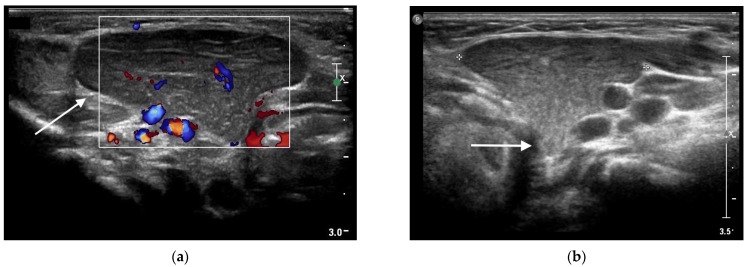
Ectopic cervical thymus in a 11-month-old toddler accidentally noticed on clinical examination for an acute upper respiratory infection. (a) Longitudinal sonogram showing a 3.5 × 1.7 cm hypoechoic lesion (*white arrow*), with intralesional vascular signs and punctate and linear echogenic foci resulting in a “speckled” or “salt and pepper” pattern, compatible with ectopic cervical thymus. (b) Portion of thymic tissue (*white arrow*) extending into deeper layers, likely connecting the ectopic mass to the orthotopic thymus.
